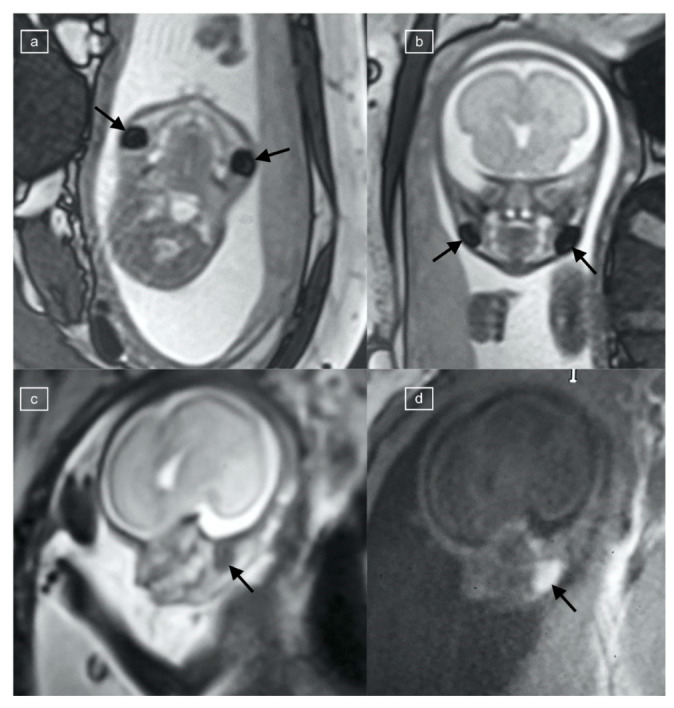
(a) Axial, (b) coronal, and (c) sagittal TRUFI MR images of a 21-week fetus, demonstrating bilateral, oval low-signal-intensity buccal fat pads (black arrows), corresponding to (d) T1 VIBE hyperintensity.
